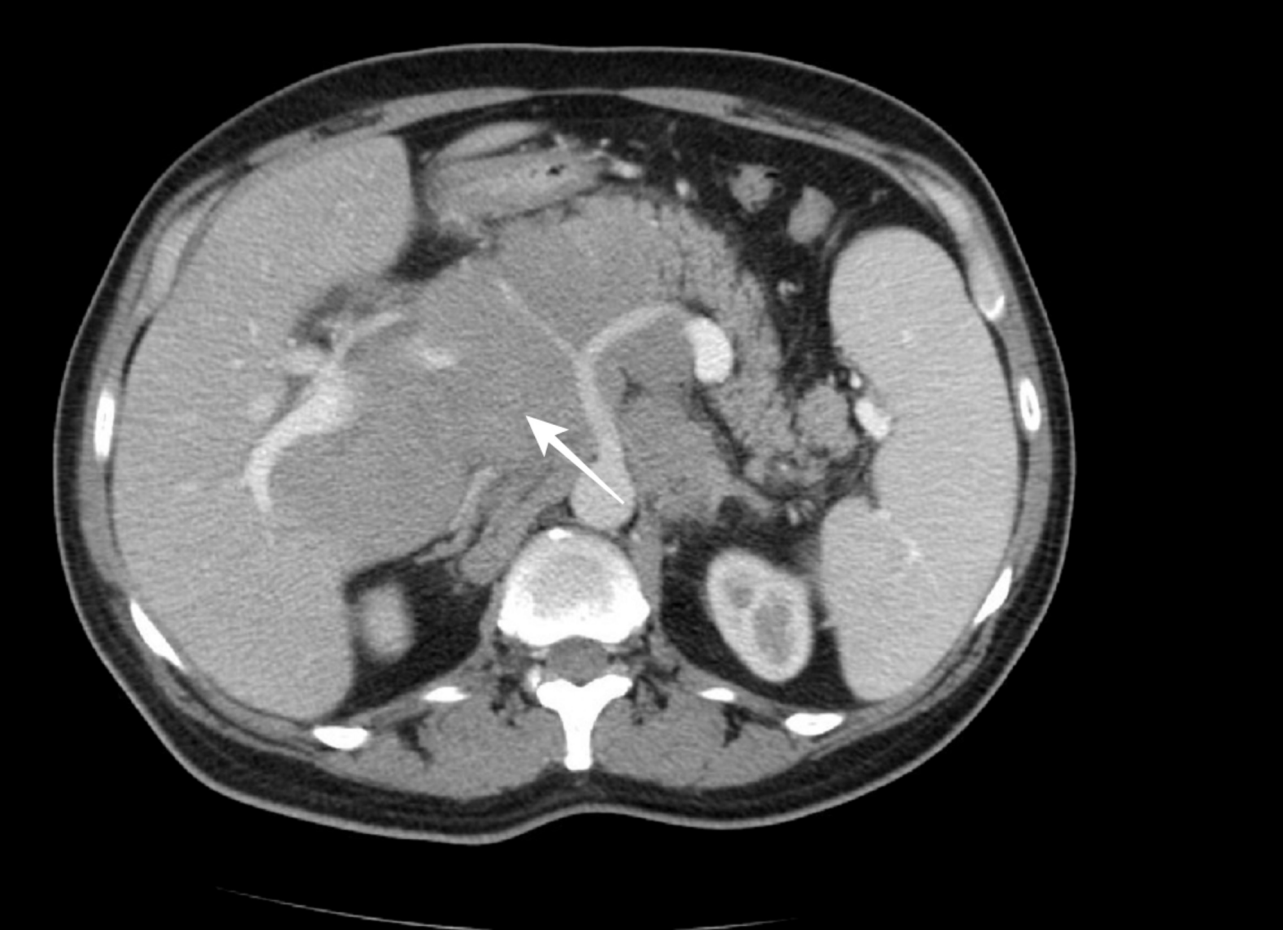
Computed tomography axial abdominal Computed tomography demonstrates extensive lymphadenopathy, resulting in a mass effect on the medial aspect of the liver.
